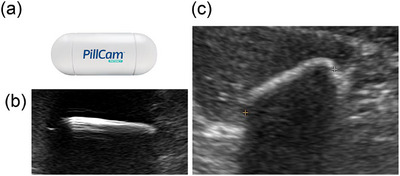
Ultrasound image of a patency capsule. (a) Patency capsule (PillCam Patency Capsule). (b) Ultrasound image of a patency capsule in water. (c) Ultrasound image of a patency capsule in the colon.
